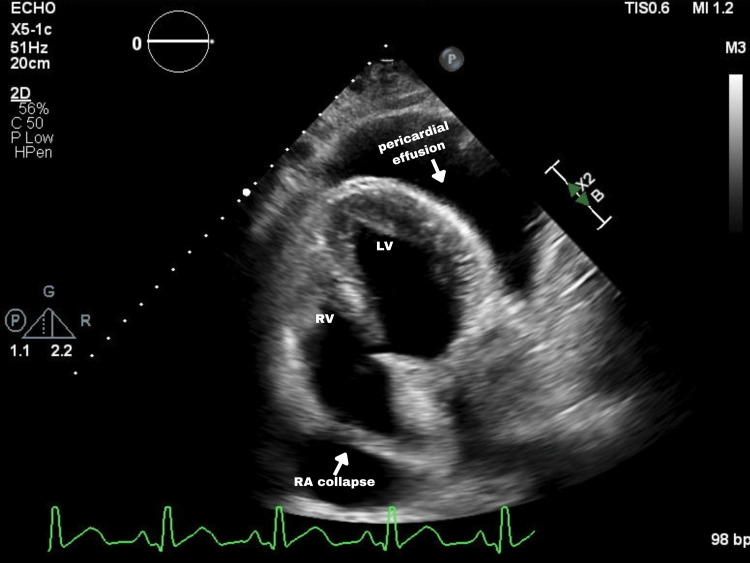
Transthoracic echocardiogram demonstrating pericardial effusion with right atrial collapse consistent with early tamponade physiology in Case 1. RA: right atrium; RV: right ventricle; LV: left ventricle
